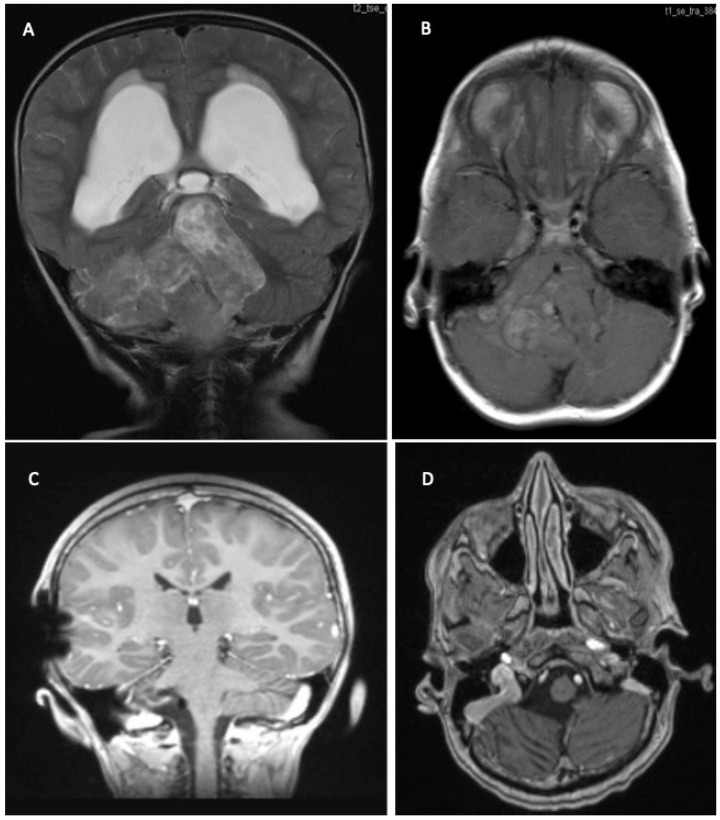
2 year old boy with psychomotor delay and signs of raised intracranial pressure. T2 (A) and contrasted T1 MRI (B) shows large posterior fossa tumor and associated hydrocephalus. Diagnosis: ependymoma without anaplasia (no molecular diagnostic) and shunt implantation. Therapy: GTR (C,D) followed by chemo- and conformal radiation therapy. Complete remission for 12 years.
